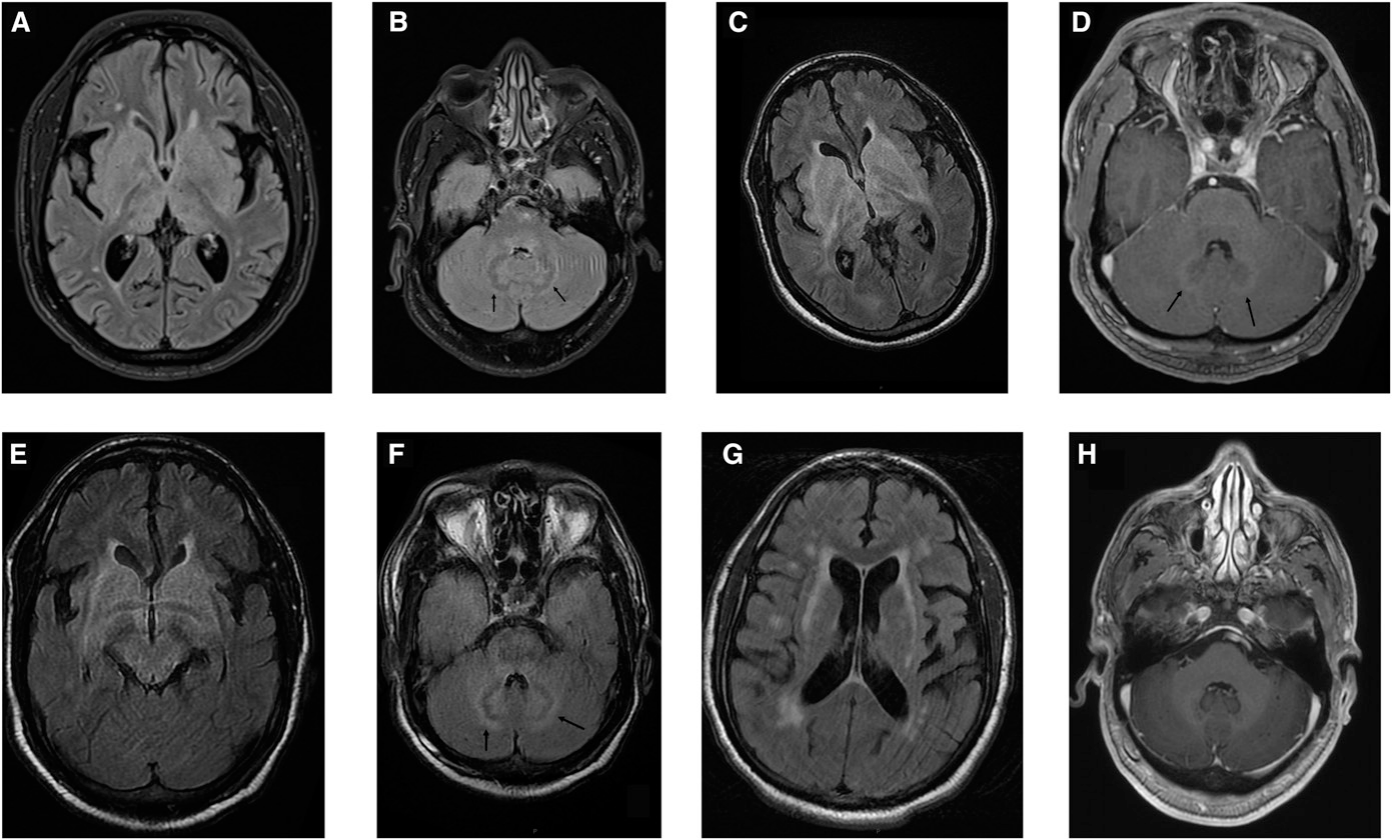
Brain MRI before and after treatment. (A and B) At admission, MRI shows on fluid-attenuated inversion recovery (FLAIR) sequence diffuse hyperintensity in both lenticular nuclei, caudate nuclei, thalami, and internal capsule (A) and hypointense signal of both dentate nuclei (DN) with a thin hyperintense blurring at the external limit of the DN (B, arrows) as well as hyperintensity at the dorsal part of the pons. (C–F) Follow-up MRI before treatment performed 7 weeks after the first MR examination demonstrates interval progression of the FLAIR hyperintensity signal in both lenticular nuclei, caudate nuclei, thalami, and internal capsule, and also of the external capsule and the hypothalamus (C). An hyperintensity on FLAIR sequence is also present in the ventral and dorsal pons and of the whole midbrain (D). Fluid-attenuated inversion recovery sequence also shows an interval increase in the hypointense signal of both DN and of the hyperintense halo (E, arrows) and areas of hyperintensity at the anterior and dorsal pons. Diffusion sequence was unremarkable. On post-contrast T1 sequence, there is an enhancement at the periphery of the DN (F arrows). (G and H) Follow-up MRI posttreatment. Follow-up MRI 1 year after initiation of treatment shows residual FLAIR hyperintensity in the external capsules. There has been new interval appearance of patchy hyperintensities of the subcortical and deep white matter, not previously observed (G). The enhancement of DN after contrast has disappeared (H).
